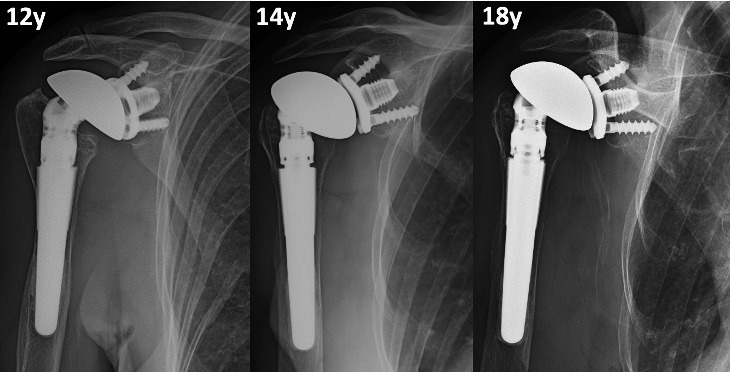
Anteroposterior radiograph views of a 61-year-old female patient with progressive wear of the polyethylene (PE) with reduced thickness between the components. At last follow-up, mild upward migration of the humeral head is observed with complete wear in the superior part of the PE.
